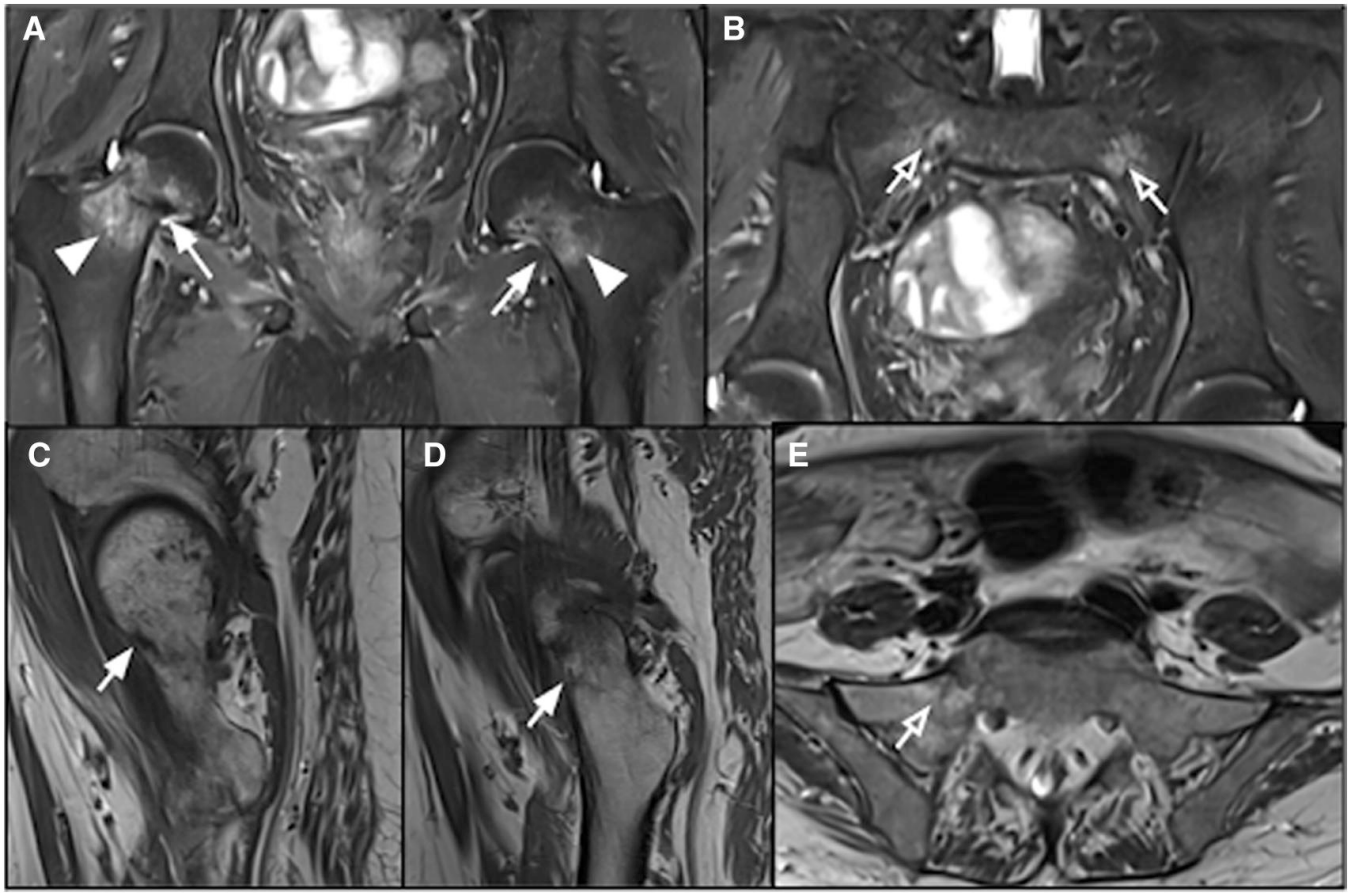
MRI pelvis showed insufficiency fractures in the femur neck and ala of sacrum on both sides—hypointense fracture line (white arrows) in neck of both femurs with surrounding marrow oedema (white arrowheads). Hypointense fracture line in both sacrum ala with surrounding marrow oedema (hollow white arrows) in coronal plane STIR image (A) and (B). Hypointense fracture line (white arrow) in neck of both femurs on sagittal plane T1W image (C) and (D). Hypointense fracture line in right sacral ala (hollow white arrow) with surrounding fat signal on axial planeT1W image (E).
